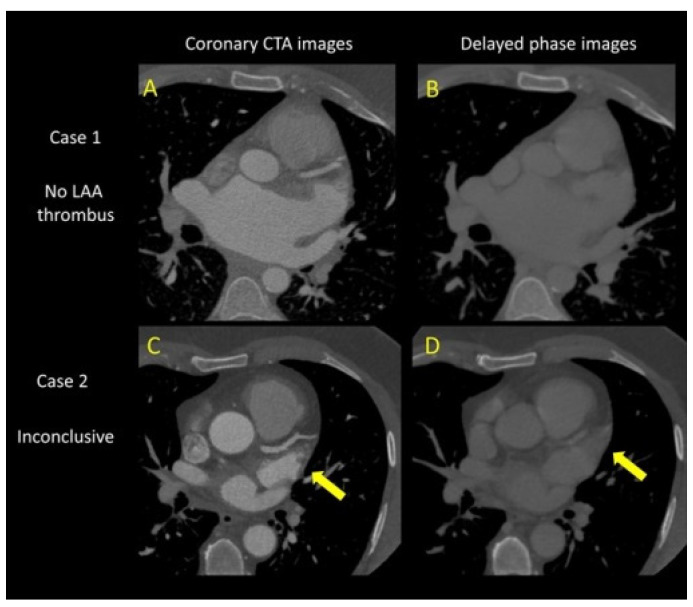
CTA image of the heart, with a yellow arrow indicating a possible left atrial appendage thrombus, which is more pronounced in the delayed-phase images. ((A) no thrombus Coronary CT, (B) no thrombus Delayed phase, (C): inconclusive for thrombus coronary CT, (D): inconclusive for thrombus Delayed phase).
